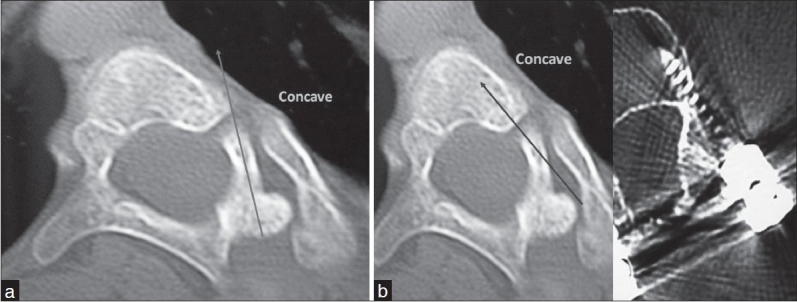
(a) Curved pedicle at the apical vertebra.Trans-pedicular screws perforate the lateral wall of the body. (b) In-out-in technique for placement of screws within the vertebral body; Post-op CT of in-out-in screw
